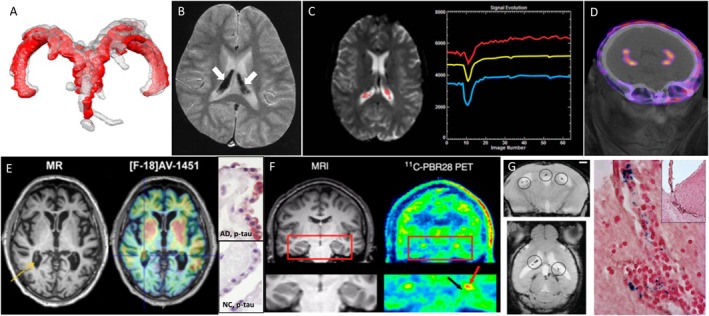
Examples of different clinical imaging techniques available for investigating the involvement of ChPs in brain disorders. (A) 3D reconstruction of ChP located within lateral ventricles space [red, before lumbar puncture (LP), and gray, after LP] in a patient with idiopathic intracranial hypertension (Lublinsky et al., 2018); (B) ChP iron deposition (arrows on hypointense signals) detected by susceptibility-weighted MRI in a patient who had received both ultrasmall superparamagnetic particles of iron oxide (USPIOs) 2 years earlier and multiple blood transfusions since (Daldrup-Link, 2017); (C) signal-time curves extracted in the ChP from dynamic susceptibility contras-enhanced MRI data: the yellow curve represents the mean signal calculated from all the pixels in the ChP volume, while the blue and red curves represent, respectively, the curve with lowest and highest baseline. After first passage of the gadolinium bolus (signal drop), the choroidal signals visually exceed the baseline which indicates gadolinium chelate extravasation into the ChP stroma (Bouzerar et al., 2013); (D) PET/CT images showing the normal ChP uptake of [^68^Ga]-DOTA-E-[c(RGDfK)]_2_ targeting α_v_β_3_ integrin (*This research was originally published in JNM.*
